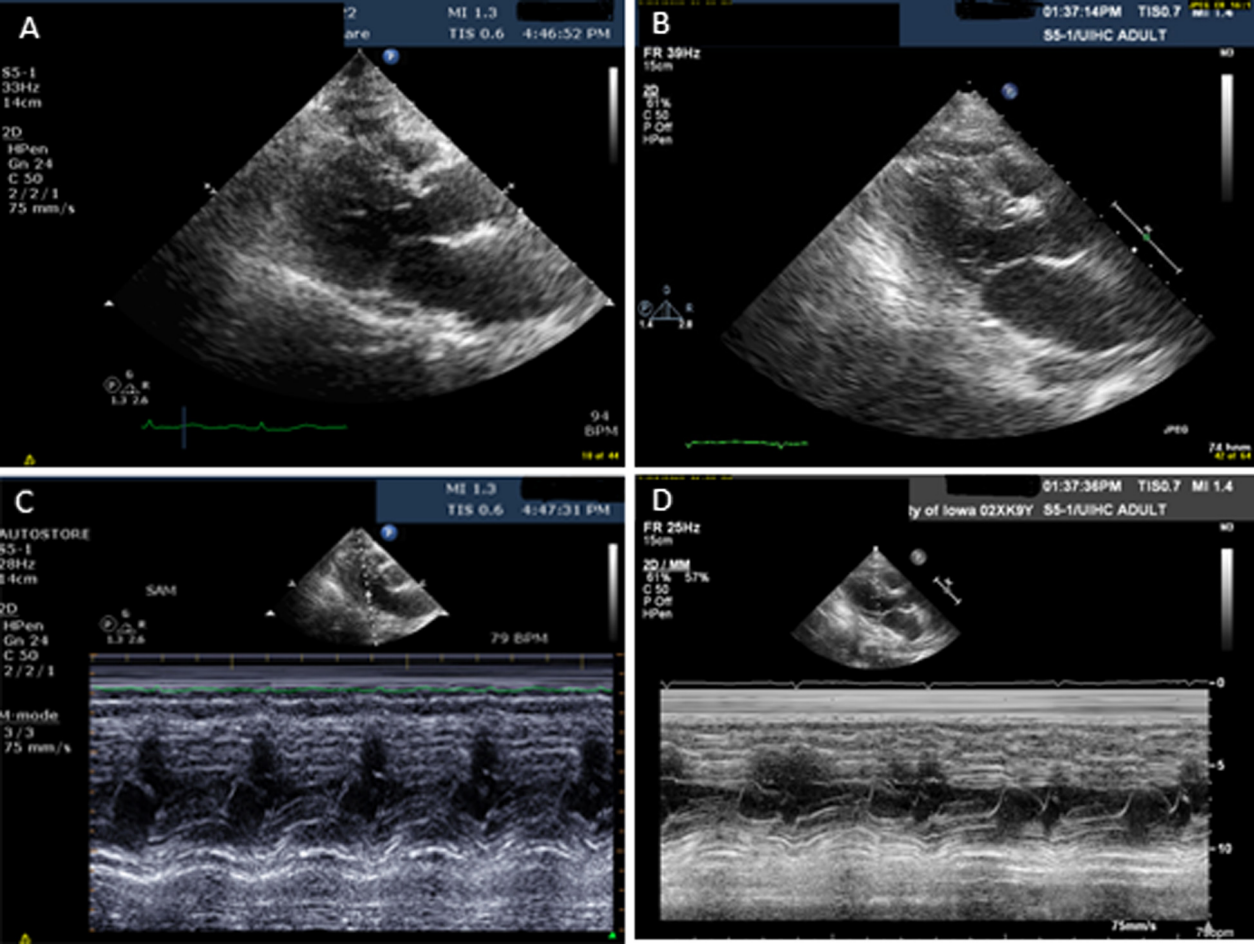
Parasternal long-axis view during systole and mitral valve M-mode. (A) Parasternal long-axis view during systole - immediate postoperative period. (B) Parasternal long-axis view during systole - postoperative day 5. (C) Mitral valve M-mode - immediate postoperative period. (D) Mitral valve M-mode - postoperative day 5.
